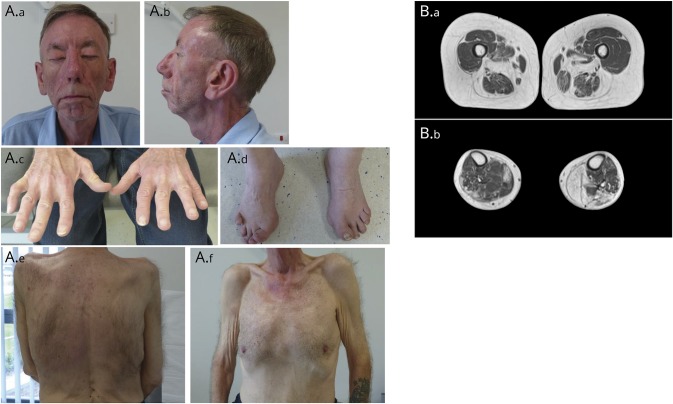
Clinical features and muscle MRI in *MYMK*-related Carey-Fineman-Ziter syndrome (A.a and A.b) Front and profile facial photographs demonstrating lagophthalmos (A.a, patient attempting lid closure), muscle hypoplasia, retrognathia, and broad nasal tip. (A.c and A.d) Wasting of intrinsic hand muscles and contracture deformities of the right little finger and the toes. (A.e, A.f) Scoliosis (A.e) and generalized muscle atrophy with pectoralis muscle hypoplasia (A.f). (B) T2-weighted MRIs of the thighs (B.a) showing severe fatty replacement of hamstrings, thigh adductors, and sartorius muscles, with relative sparing of the gracilis and quadriceps muscles bilaterally, and of the calves (B.b) showing asymmetric involvement with more marked fatty replacement in muscle of the right leg. Gastrocnemius and soleus muscles are severely affected, and the tibialis anterior on the right is relatively spared.
